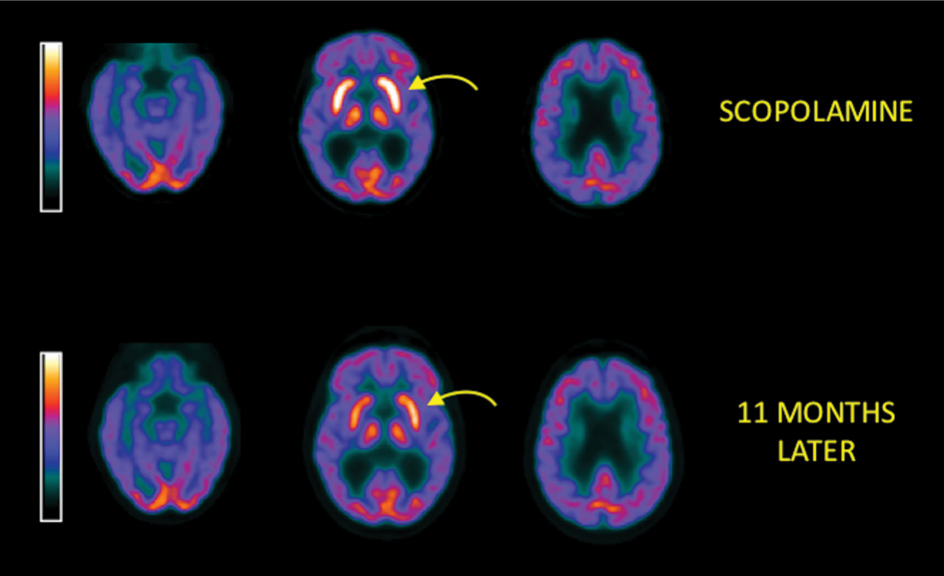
Top: Axial brain slices of a 2-deoxy-2-(^18^F)fluoro-D-glucose positron emission tomography (FDG-PET) scan dated 11/30/10: left slice = level of temporal lobes, middle = basal ganglia, right = frontal and parietal cortex. Scan shows markedly increased tracer uptake in the basal ganglia (arrow indicates left striatum), which appears striking against the normal uptake diffusely in the cortex. Basal ganglia to cortex ratio = 2.1. This finding has not been described consistently for any neurological disorder and is presumably secondary to scopolamine. Color bar indicates standardized uptake value (SUV) and runs from 0 (black) to 14 (white). On this display scale, the basal ganglia are actually “burned out” bright, with an SUV of 20. Bottom: FDG-PET performed on 10/25/11 demonstrating essentially normal tracer uptake in the basal ganglia (arrow) and cortex. The basal ganglia to cortex ratio = 1.5. This represents a dramatic improvement from the previous scan. The striking interval improvement in the scan supports the hypothesis that the original PET abnormality was a medication effect. Color bar indicates SUV and runs from 0 (black) to 14 (white).
